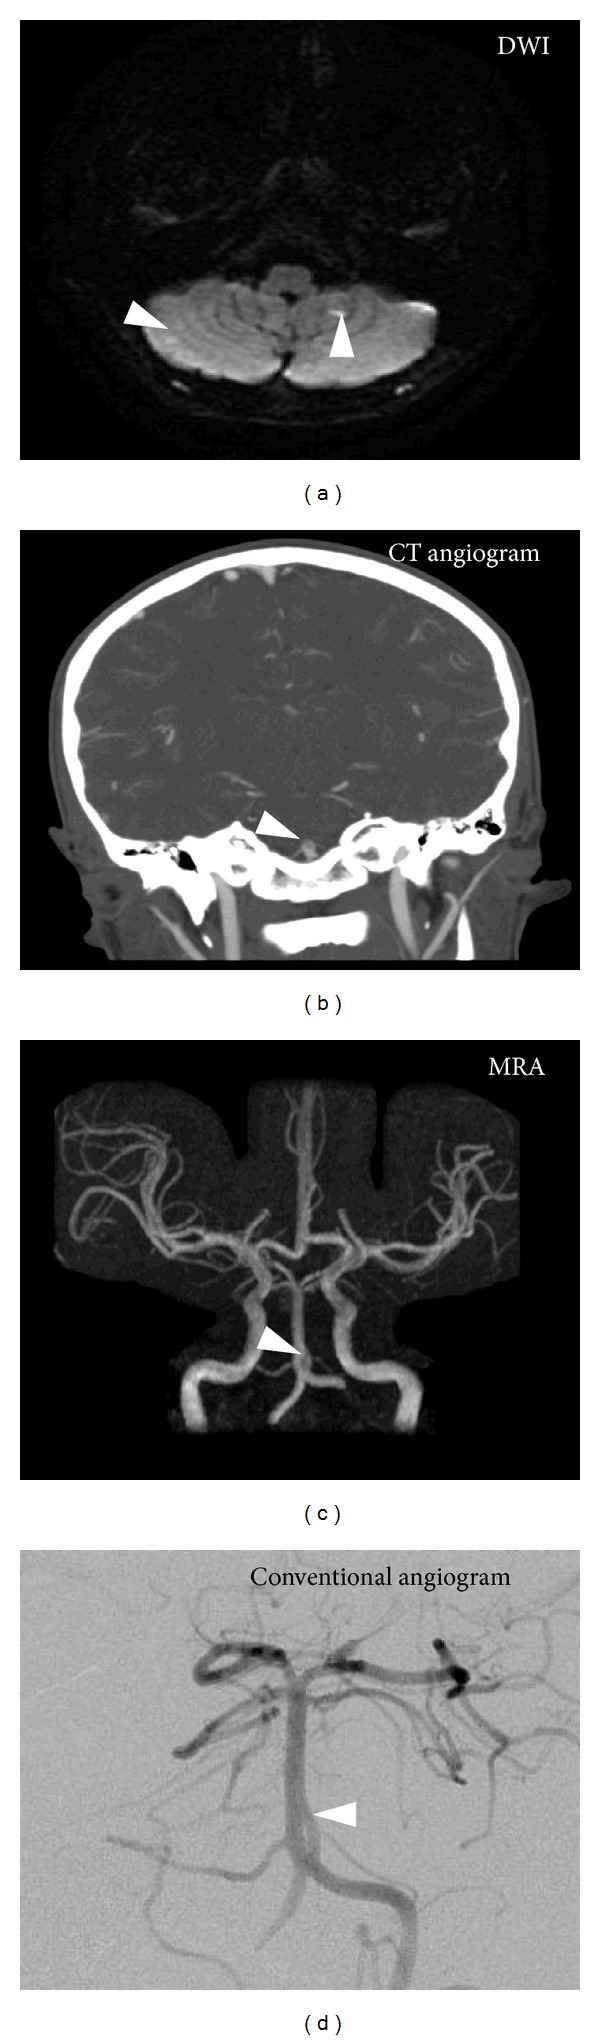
(a) Diffusion-weighted MRI sequence reveals punctate areas of reduced diffusivity in bilateral cerebellar hemispheres (arrowhead). (b) CT angiography and (c) MR angiography reveal an area of signal abnormality of the basilar artery at the level of the anterior inferior cerebellar artery (arrowhead). (d) Conventional angiography confirmed the area of abnormality as a type 2 basilar artery fenestration (arrowhead) without evidence of dissection, thrombus, or aneurysm.
